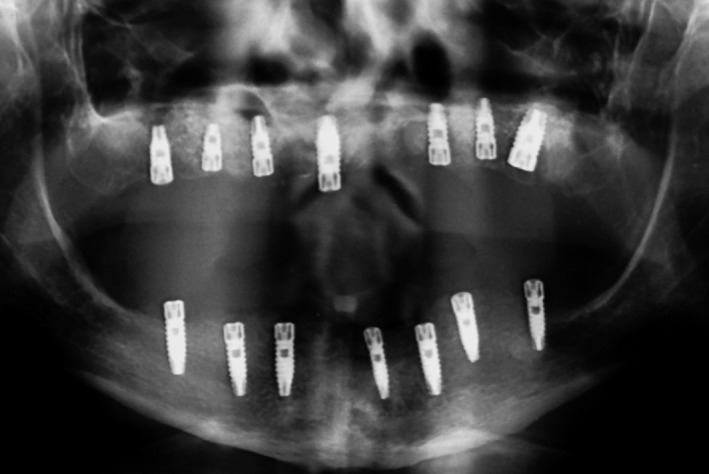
Panoramic radiograph of seven implants in each arch.
